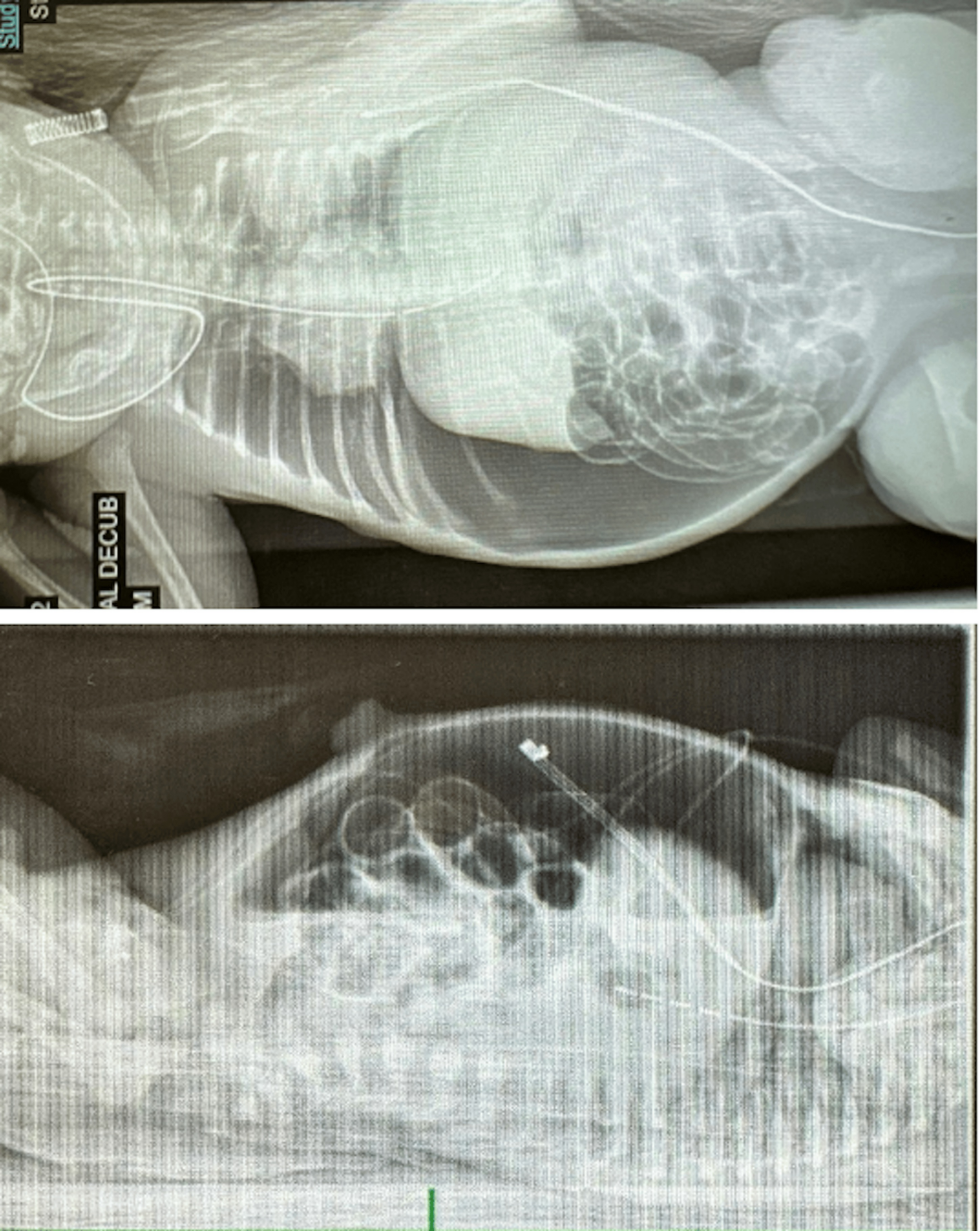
X-rays taken at day one of life indicating massive pneumoperitoneum Supine and decubitus abdominal radiographs obtained on day one of life demonstrate large-volume pneumoperitoneum with bowel loops outlined by free intraperitoneal air and displaced centrally. While intraluminal bowel gas is present, there is no radiographic evidence of pneumatosis intestinalis or portal venous gas.
